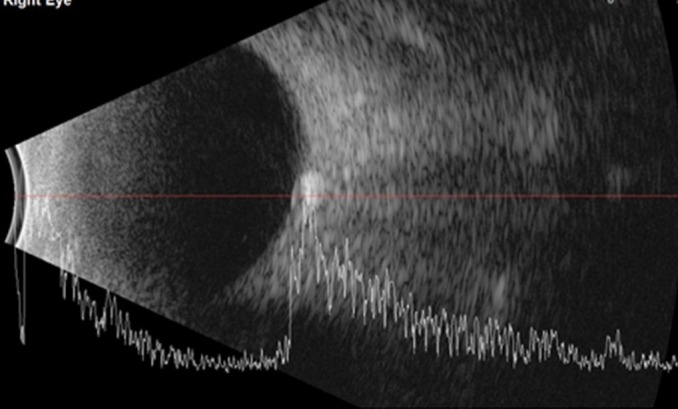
B-scan ultrasonography in the right eye
